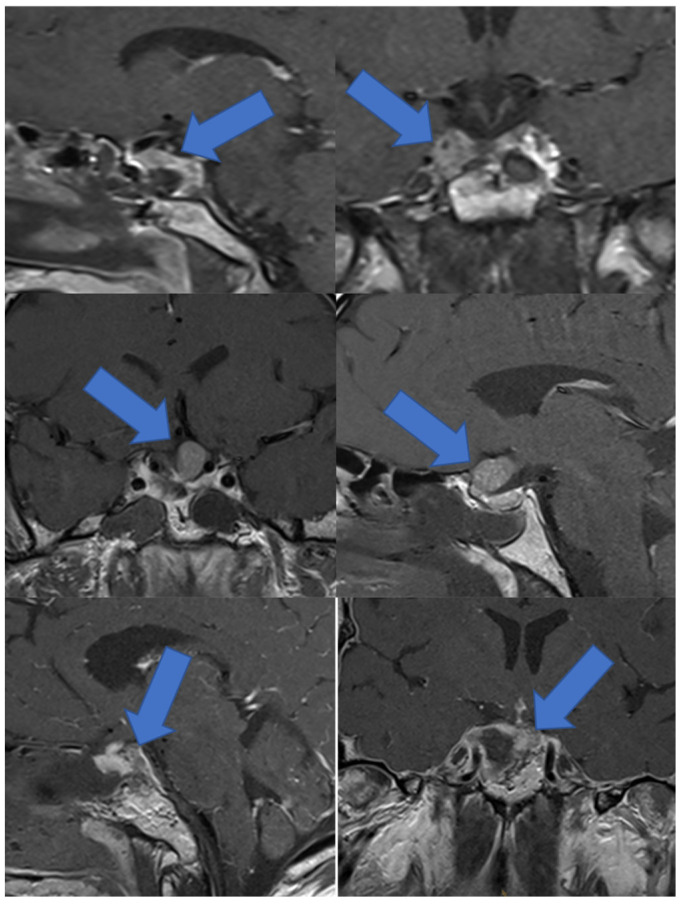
Pictures in the first row depict sagittal and coronal- with gadolinium-enhanced MRI, showing intracavernous tumor remnant. In the second row, tumor remnant perforating the diaphragm is depicted on coronal- and sagittal-enhanced MRI scans, and in the third row, adenoma remnant infiltrating the clivus is shown.
